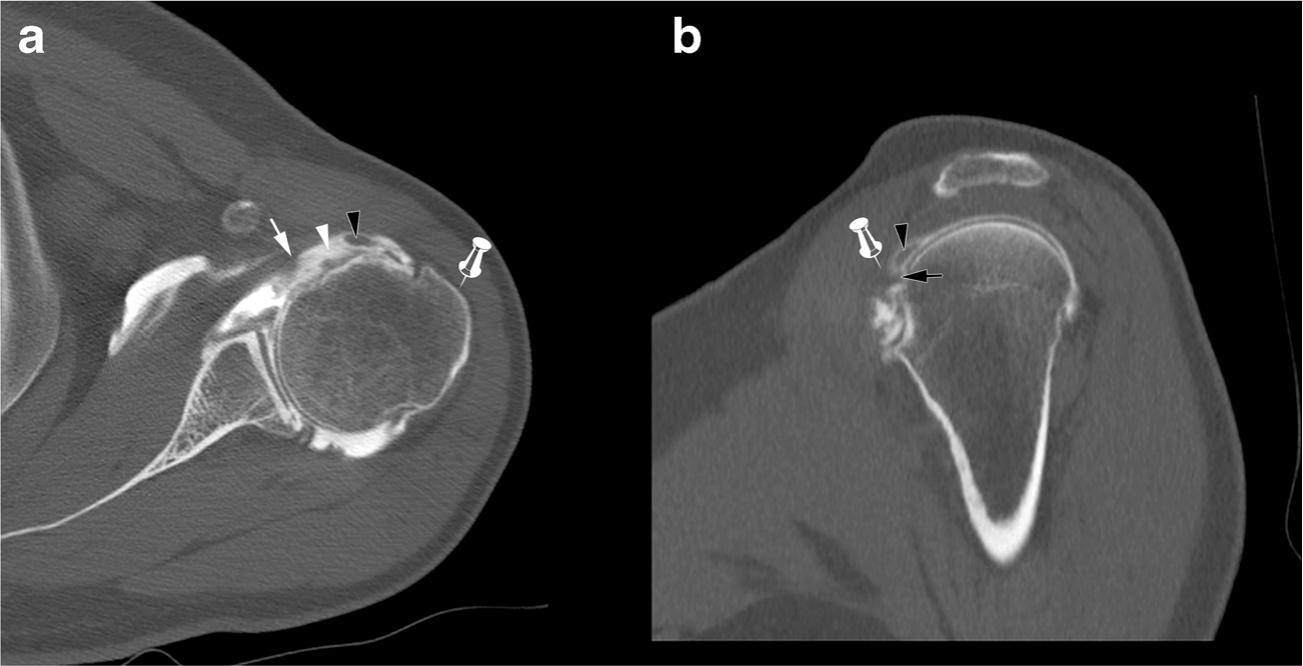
Forty-five-year old man with pain of the anterior shoulder. Computed tomography arthrography of the left shoulder with axial (a) and sagittal oblique (b) reconstruction. Complete partial thickness tear of the SSC tendon as shown by the absence of opacification of SASD bursa (pin), and the whole disruption of the tendon in the lateral direction (arrow head). The tendinous stump presents an intermediary retraction (arrow). This tear leads to a medial subluxation of the LHBT (black arrowhead) (a). Note that an intact superior glenohumeral ligament (black arrow) prevents the LHBT from inferior dislocation (b)
